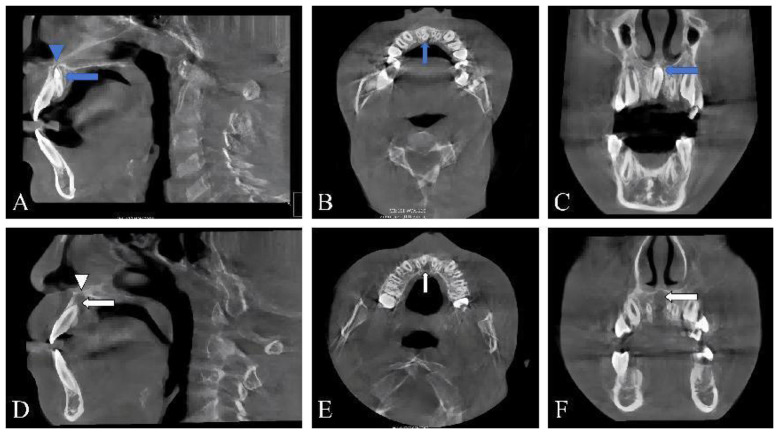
Preoperative and postoperative CBCT images of the inverted supernumerary tooth in the maxilla. (A–C) Preoperative images clearly demonstrate the location of the supernumerary tooth (indicated by blue arrows). (A) Sagittal view shows perforation of the hard palate by the tooth crown (indicated by a blue triangle). (B) Axial view reveals the close proximity between the root of the supernumerary tooth and the adjacent tooth root. (C) Coronal view confirms the completely inverted orientation of the tooth. (D–F) Postoperative images confirm the complete removal of the supernumerary tooth. The original site is now visible as a well-defined extraction socket (indicated by white arrows). A corresponding bony defect is evident at the site of the previous palatal perforation (indicated by a white triangle).
